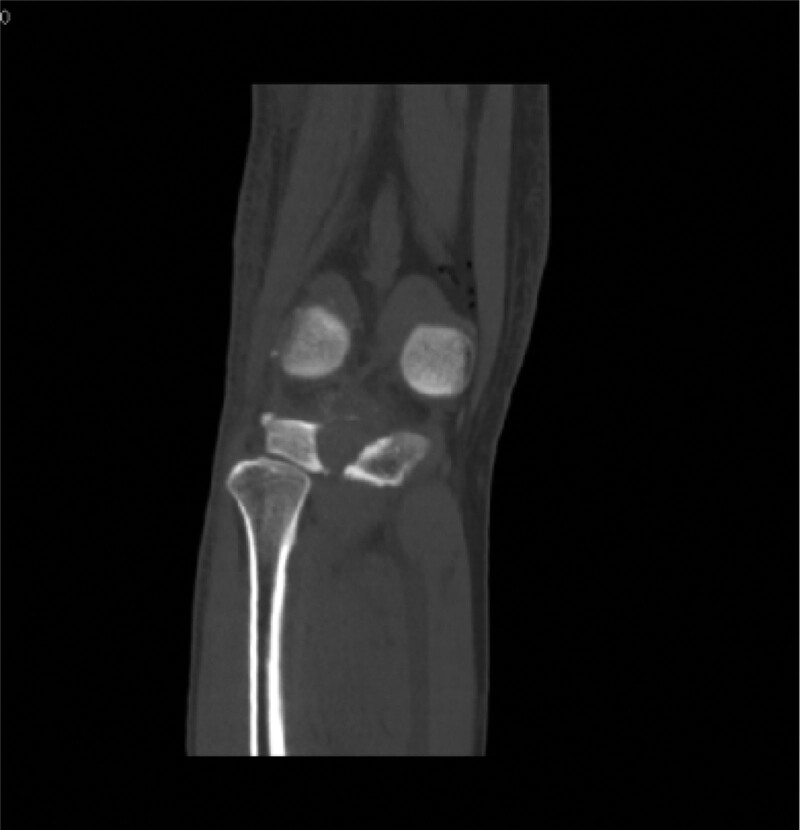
Postoperative CT image in coronal plane. CT = computed tomography.
